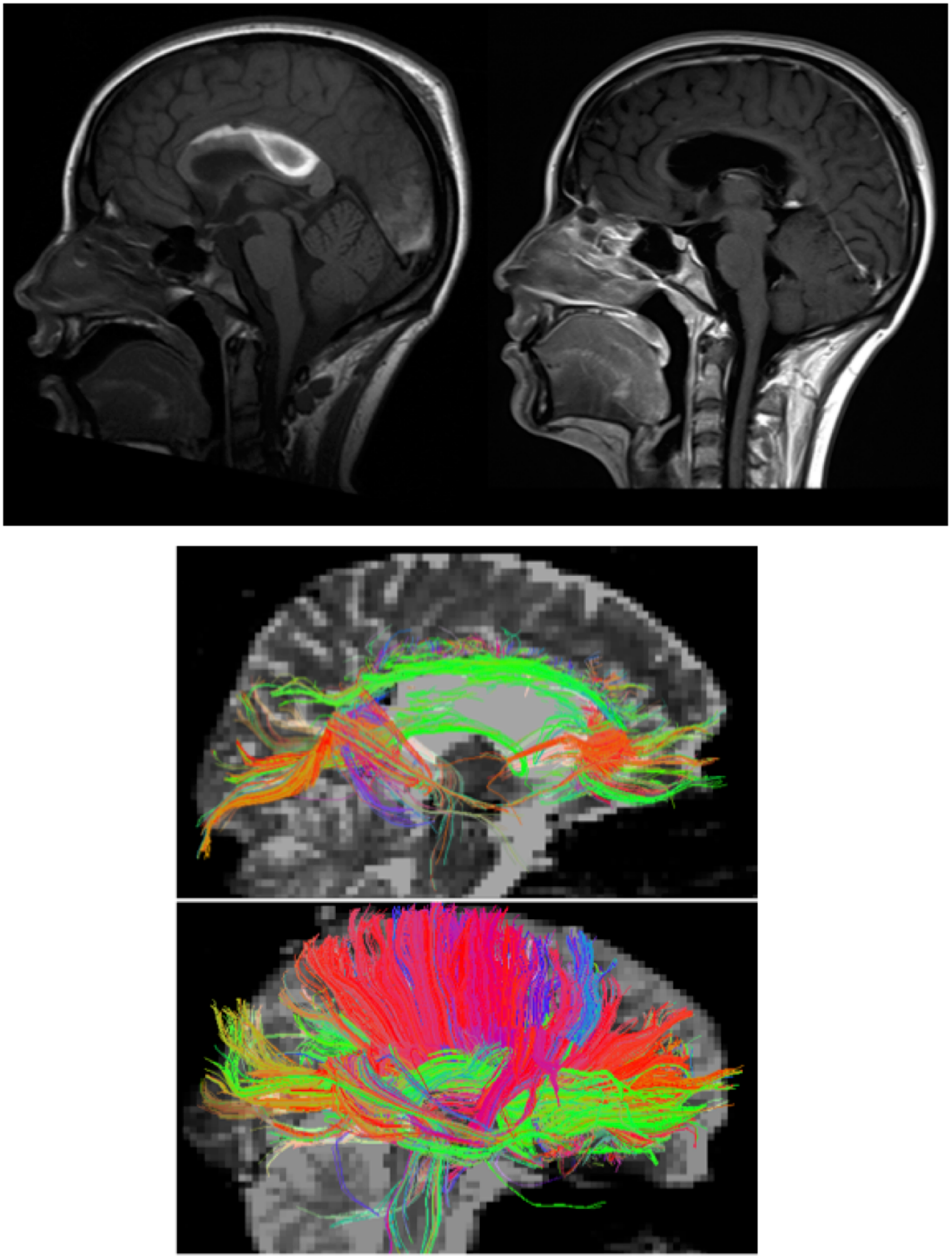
Top*. Sagittal T1 MRI brain images of patient. Left: day 17, showing a large callosal haematoma. Bilateral subdural haematomas were also present as were post-surgical changes related to the previous ganglioglioma resection with ex-vacuo dilatation of the left temporal lobe. However there was no evidence of diffuse axonal injury and no evidence of cortical damage. Right: 12 months later, showing resolution of the haemorrhagic changes but marked atrophy of the body of the corpus callosum. MRI brain at 20 months showed no further changes (not shown). *Bottom*. Deterministic tracking of the callosal tract based on two regions of interest defining the corpus callosum on sagittal planes to the left and right of midline. The patient is above with a control below for comparison. The majority of interhemispheric direct connections (red) are lost except at the extremes of the genu and splenium
